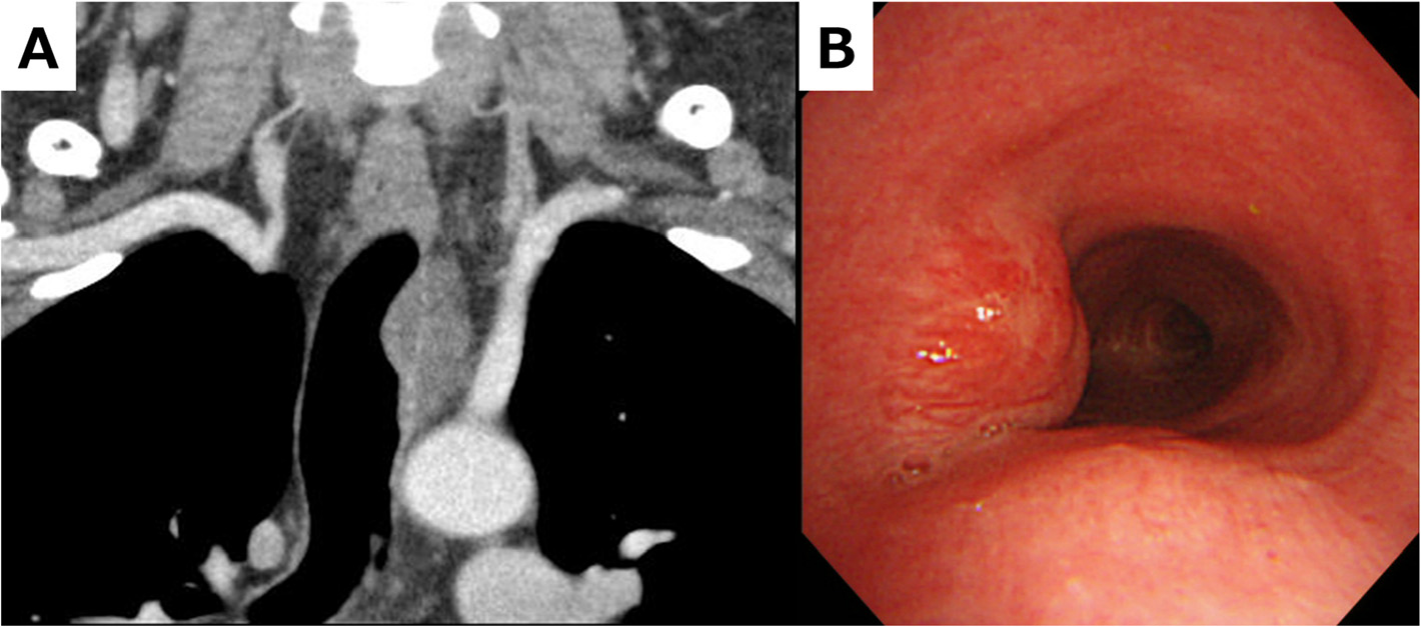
Preoperative findings. a Coronal view of chest computed tomography (CT). A tumor involved 3.5 cm of the left tracheal wall, protruding inside and outside the tracheal wall. b Bronchoscopy showing a submucosal tumor affecting four tracheal cartilaginous rings
